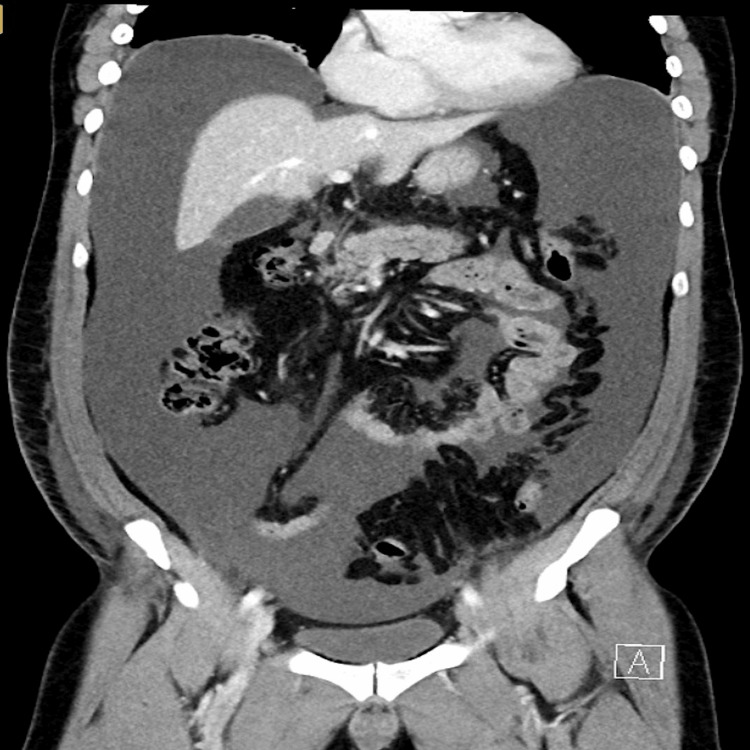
Abdominal computed tomography on postoperative day 23 showed marked four-quadrant ascites.
